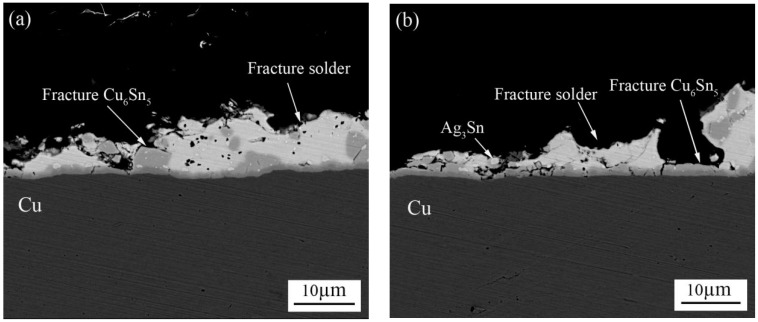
Fracture modes in solder joints formed at an 800-ms ultrasonic bonding vibration time for aging times of (a) 240 h and (b) 360 h.
